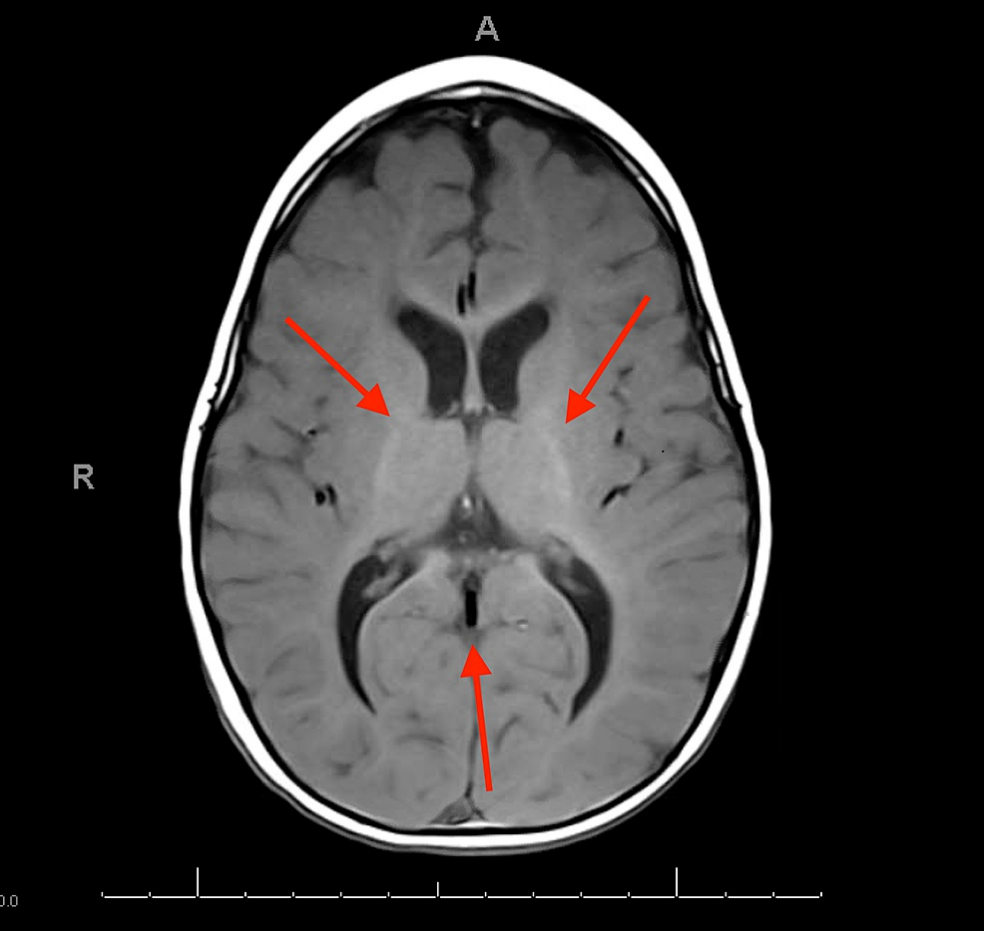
MRI of the brain revealing incomplete myelination with decreased white matter volume and a relatively thin volume of the corpus callosum with ex-vacuo prominence of the ventricles, cisterns, and sulci.
